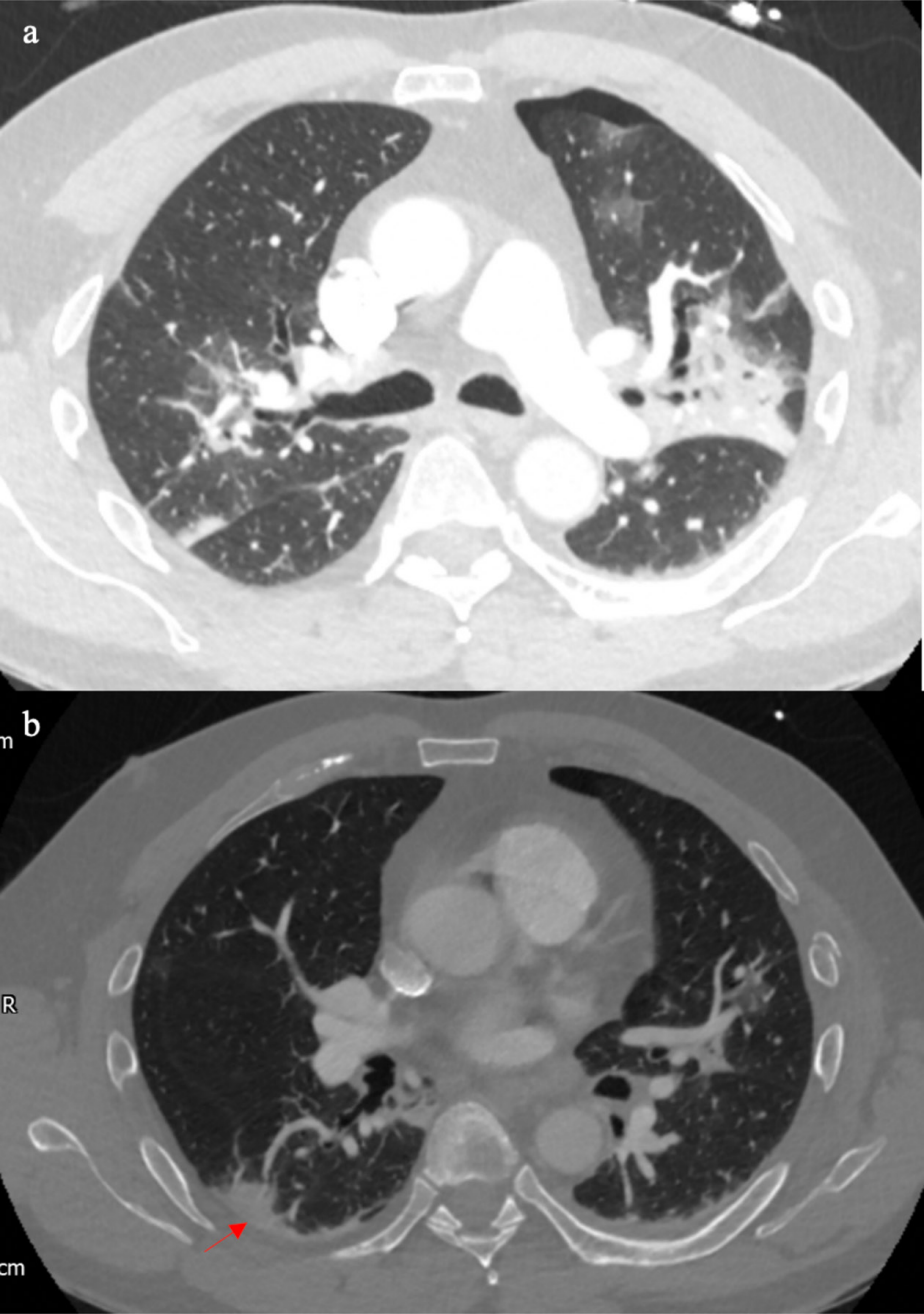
Patient with fall onto left side and trauma CT showed left-sided pneumothorax with associated left-sided rib fractures (10a–b). The centrally affected areas particularly those in the right lung were most likely from COVID-19 infection.The right posterior peripheral consolidation (arrow) could be from contre-coup injury, but infective consolidation was also possible given the absence of subpleural sparing for acute contusion.
